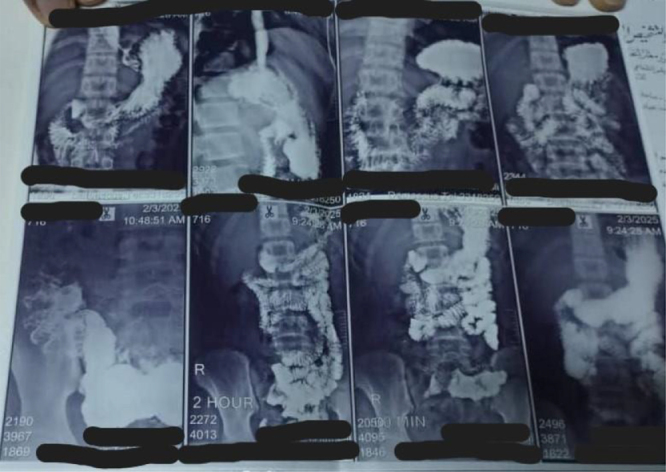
CT scan – contrast-enhanced abdominal CT in the coronal plane demonstrating the reduced aortomesenteric angle.
